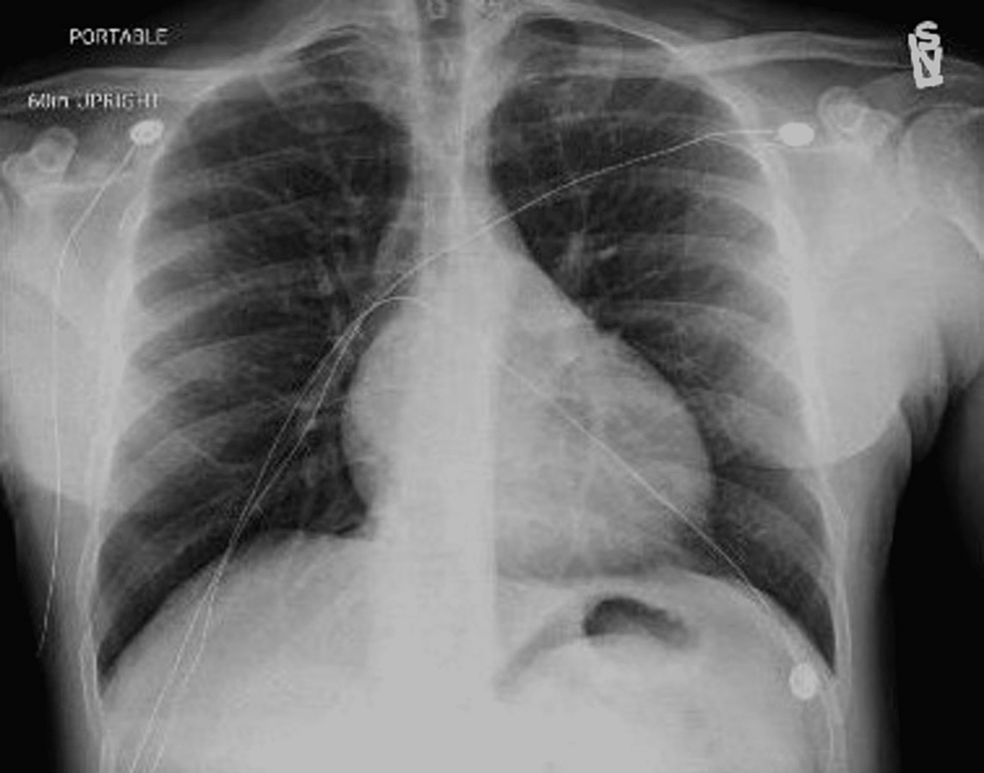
Chest x-ray showing normal cardiac silhouette and lung fields
